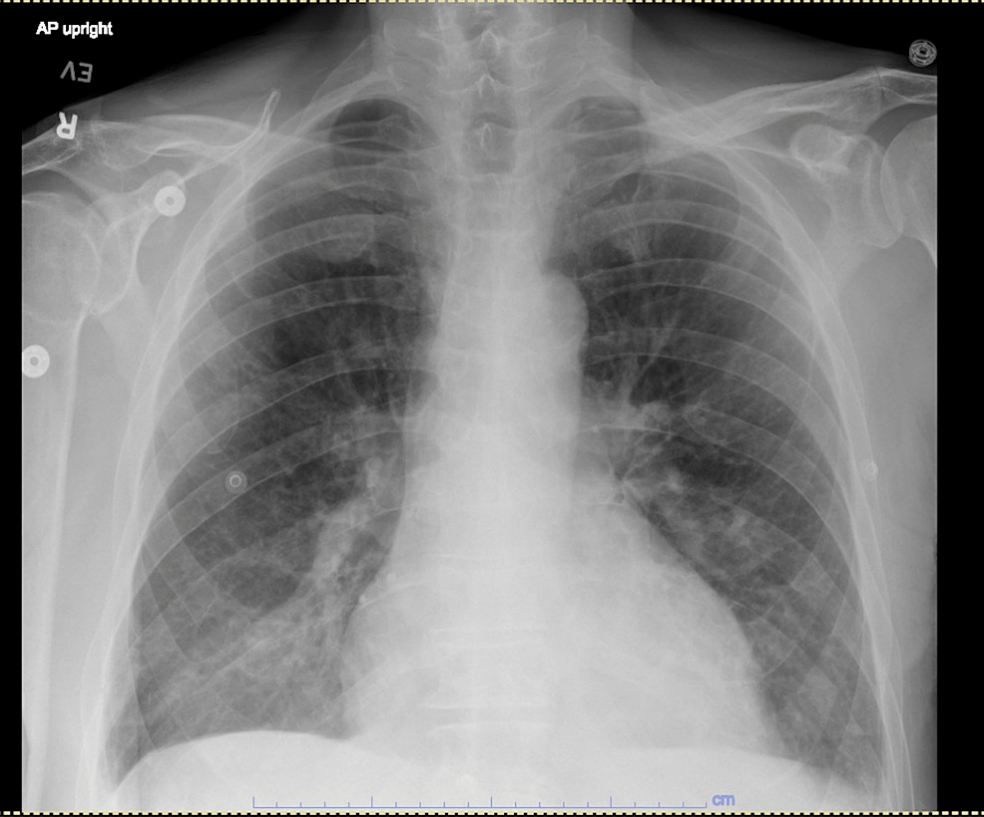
Chest X-ray Bilateral opacities were noted, most prominent on the right side
